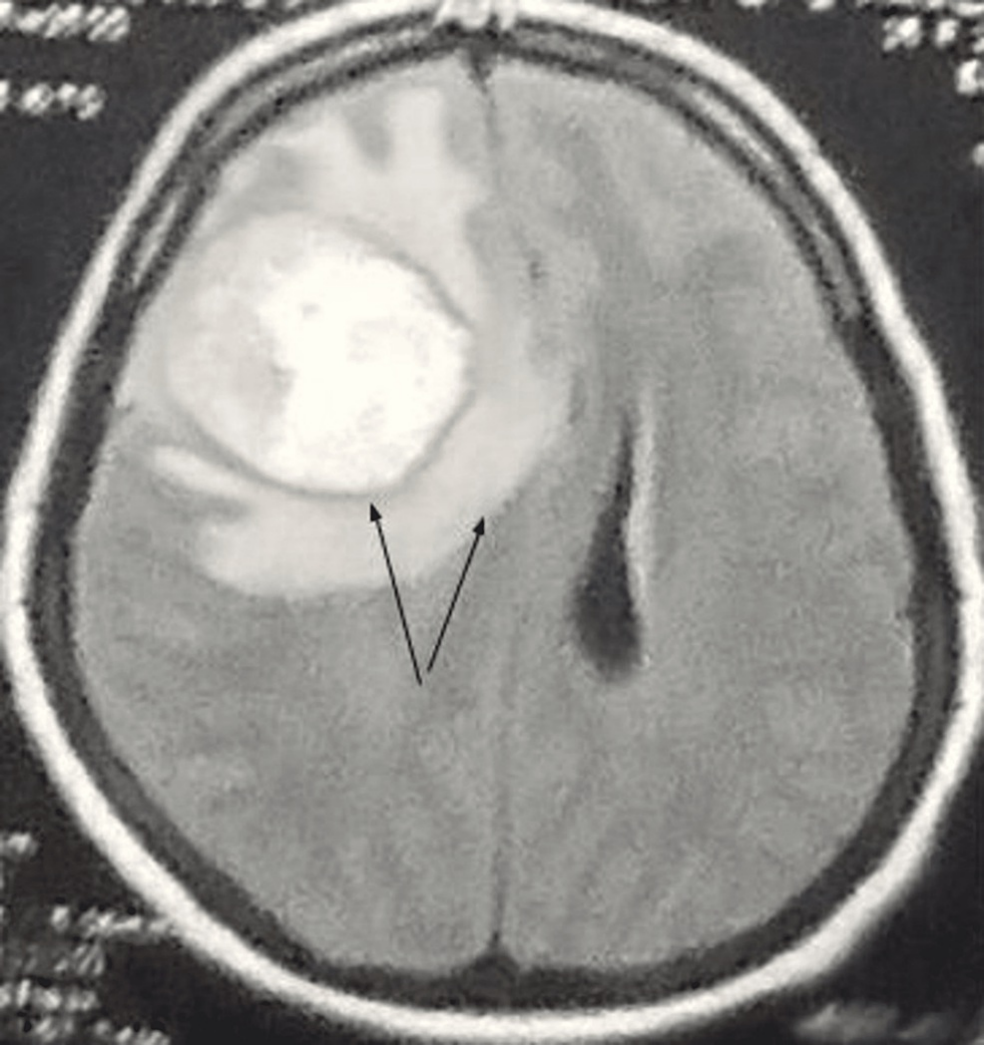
Axial MRI of the brain (FLAIR-weighted sequence) demonstrating lesion in the right frontal lobe with perilesional edema (arrow). The mass effect of the lesion causes a midline shift of 15mm towards the left side. FLAIR: fluid-attenuated inversion recovery
